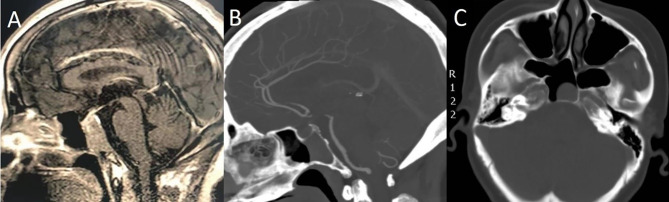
A 55-year-old woman with headache for 4 months. (A) Preoperative sagittal T1-weighted MRI shows PA with clival invasion. (B) Sagittal CT bone window shows that the patient had sufficient SS pneumatization. The cortical bone of the SS floor was intact, and the tumors were all in the SS without clival bony erosion. (C) Axial CT bone window shows that the tumor was completely located in the SS.
